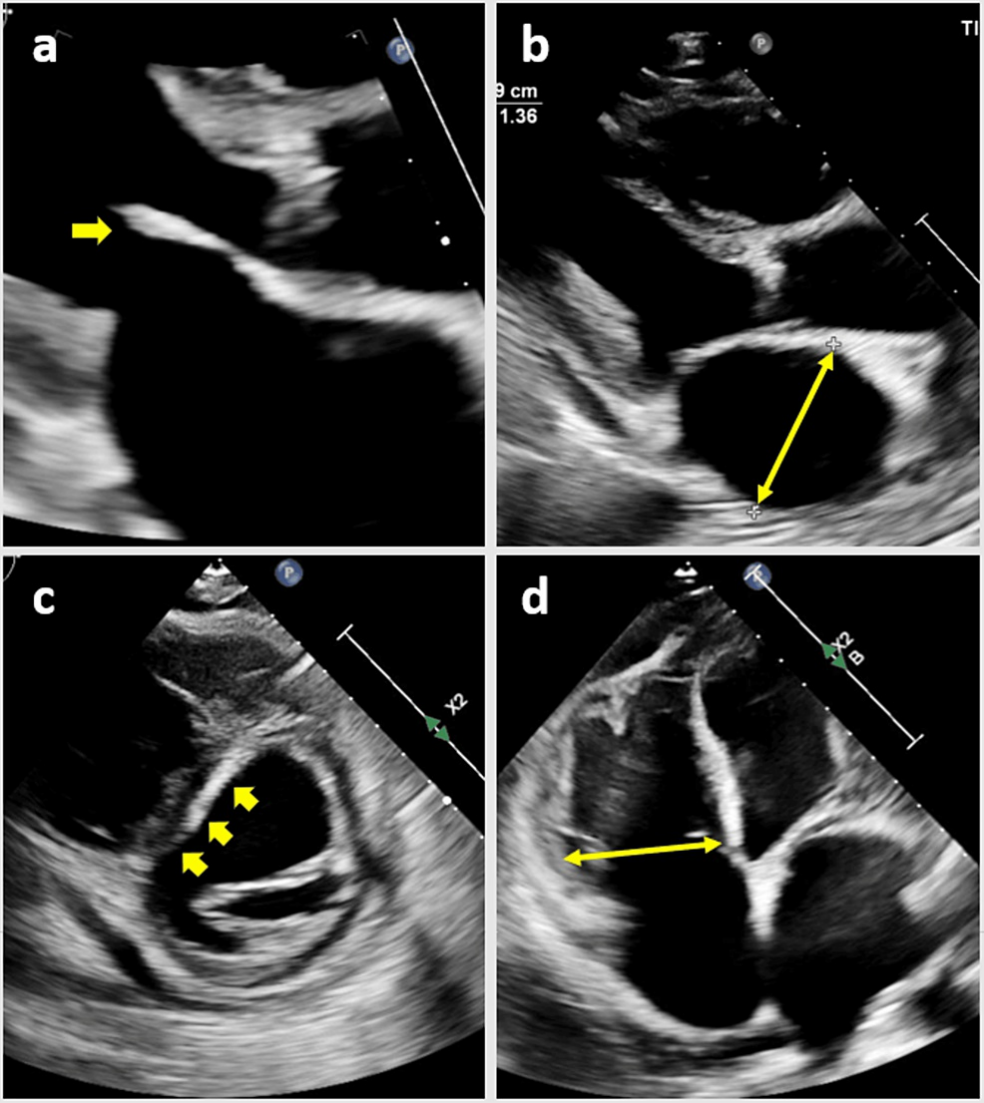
The TTE from the current hospitalization (a) Mildly thickened anterior mitral valve leaflet. (b) Severe left atrial dilation. (c and d) Interventricular septal flattening with a "D" shape configuration and severe right ventricular dilation, respectively TTE: transthoracic echocardiogram
